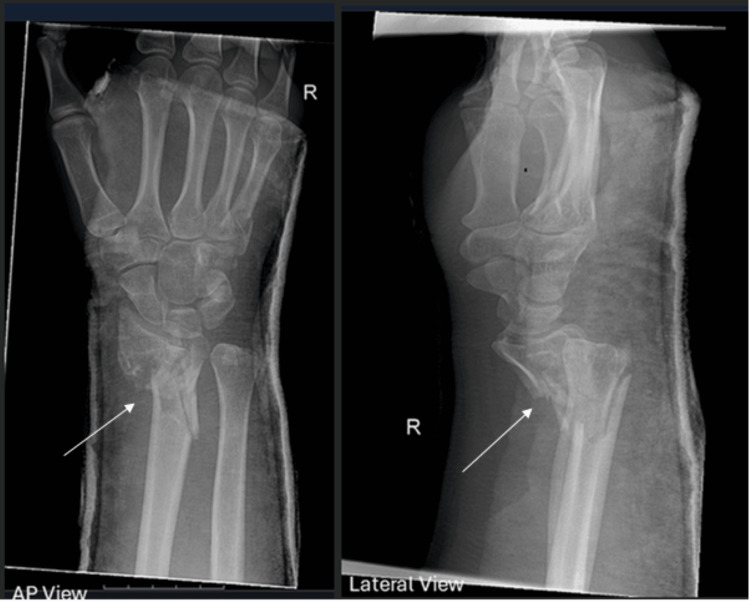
Admission X-rays of the right distal radius showing a comminuted intra-articular fracture with volar displacement (anteroposterior and lateral views). Arrows indicate fracture fragments.
